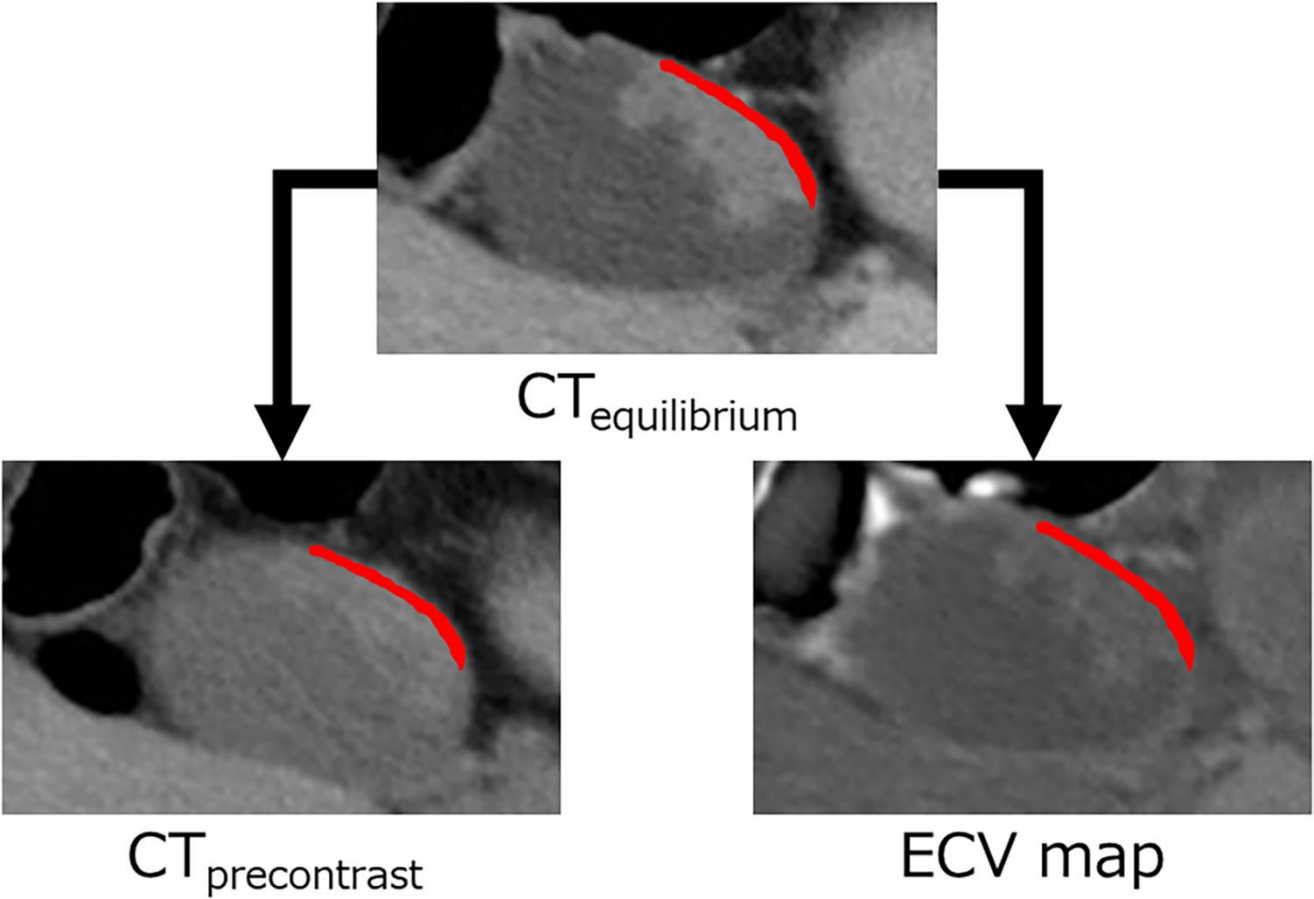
Images depicting duplicating and positioning of ROI on the gallbladder wall. The radiologists selected the maximal cross-section of GBCA on axial images, referencing equilibrium phase CT images (CT_equilibrium_), and delineated ROI on the wall where the GBCA is present, excluding perigallbladder fat and bile. The same ROIs are applied to the pre-contrast CT images (CT_precontrast_) and ECV map. Misalignments or ROIs extending outside the gallbladder wall are manually corrected. After correction, the ROIs are reviewed by the radiologist and coordinator to ensure proper positioning within the gallbladder wall. *ROI* region of interest, *GBCA* gallbladder carcinoma, *ECV* extracellular volume
